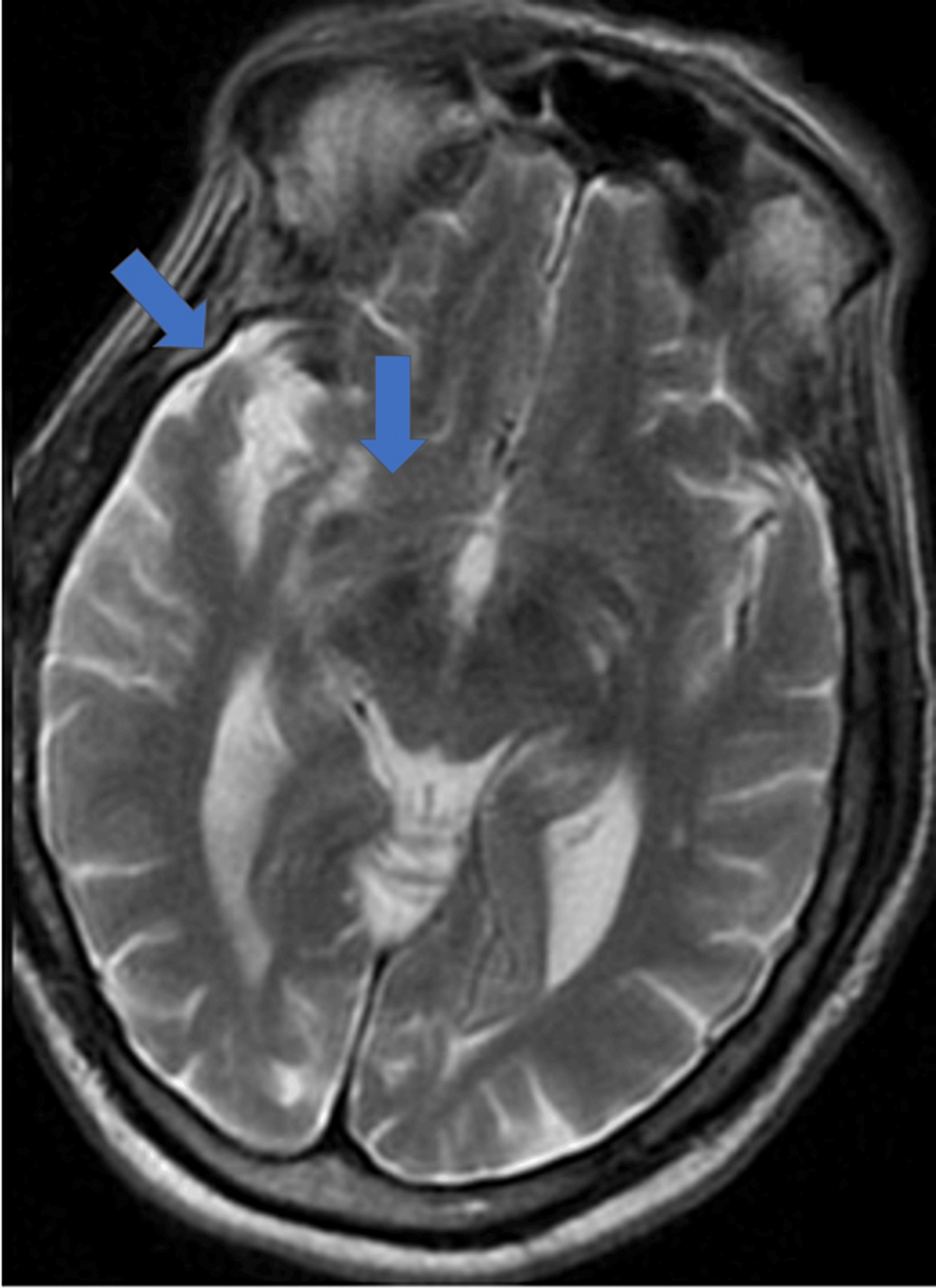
Magnetic resonance imaging without intravenous contrast was limited due to motion and was negative for stroke, mass, and intracranial bleeding. It revealed the same chronic infarct involving the lateral right basal ganglia as previously noted on head CT one week earlier.
